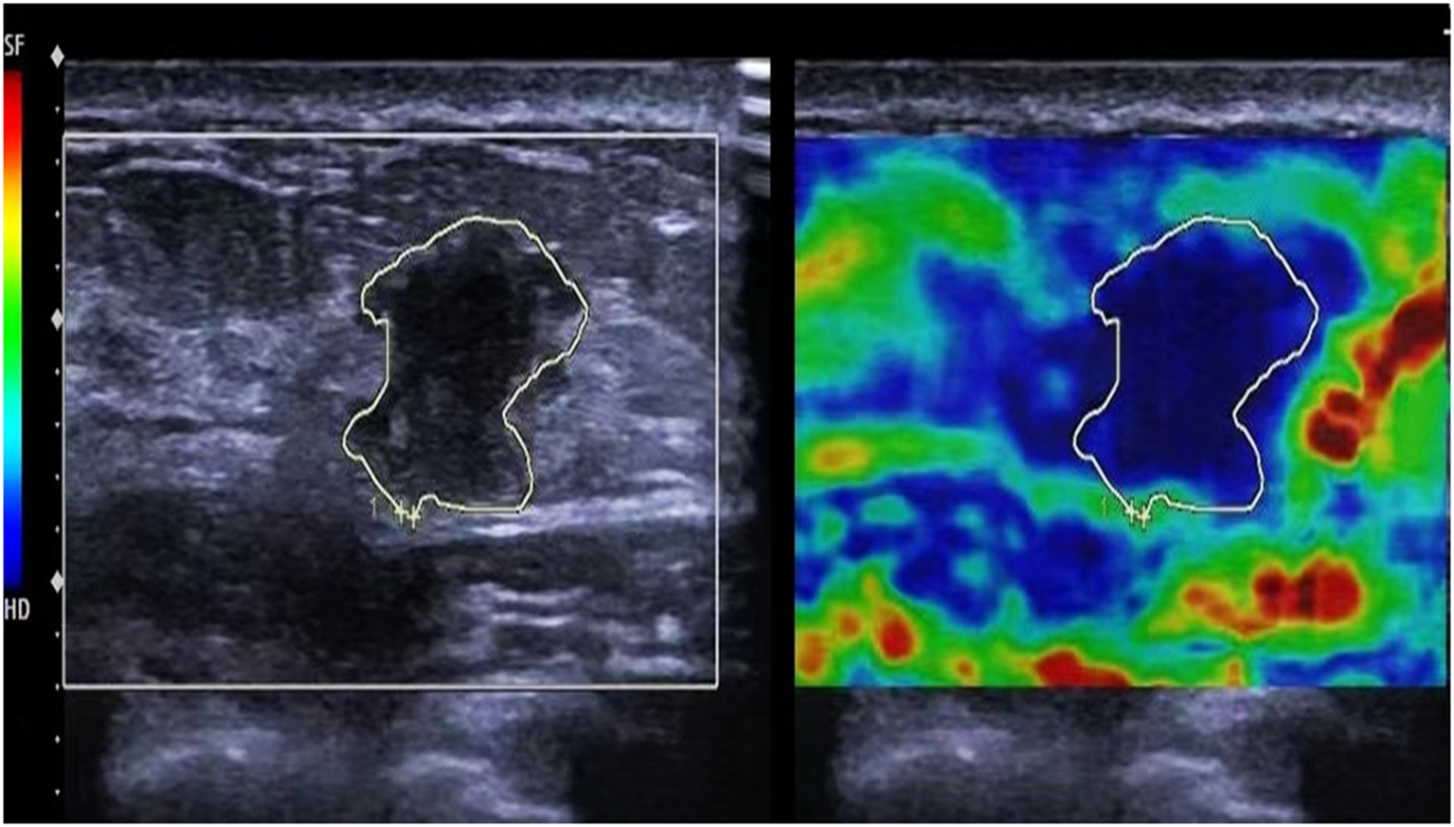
Ultrasound elastography of the patient. The tumor and surrounding tissues appearing blue with no deformation, exhibiting an ultrasound elastography elasticity score of 5.
